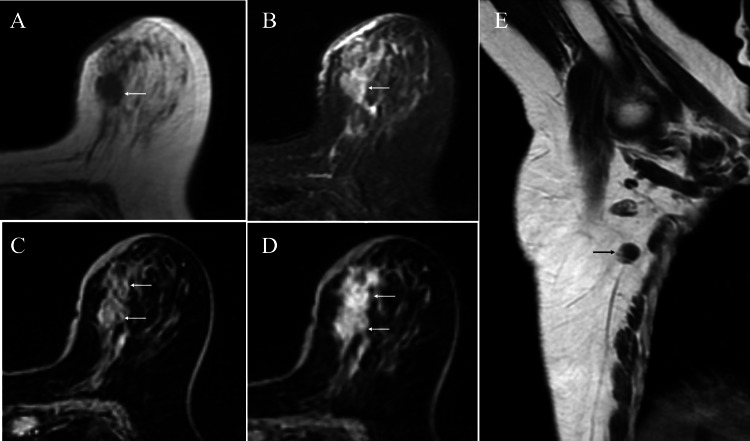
Magnetic resonance imaging (MRI) findings MRI of the tumor showed low signals on T1-weighted images (A, arrow), weak high signals on fat-suppressed T2-weighted images (B, arrow), and slow and persistent heterogeneous enhancement on the initial (C, arrows) and delayed (D, arrows) phase dynamic studies, respectively. Magnetic resonance axillography showed eccentric cortical hypertrophy (E, arrow) in one normal-sized lymph node.
